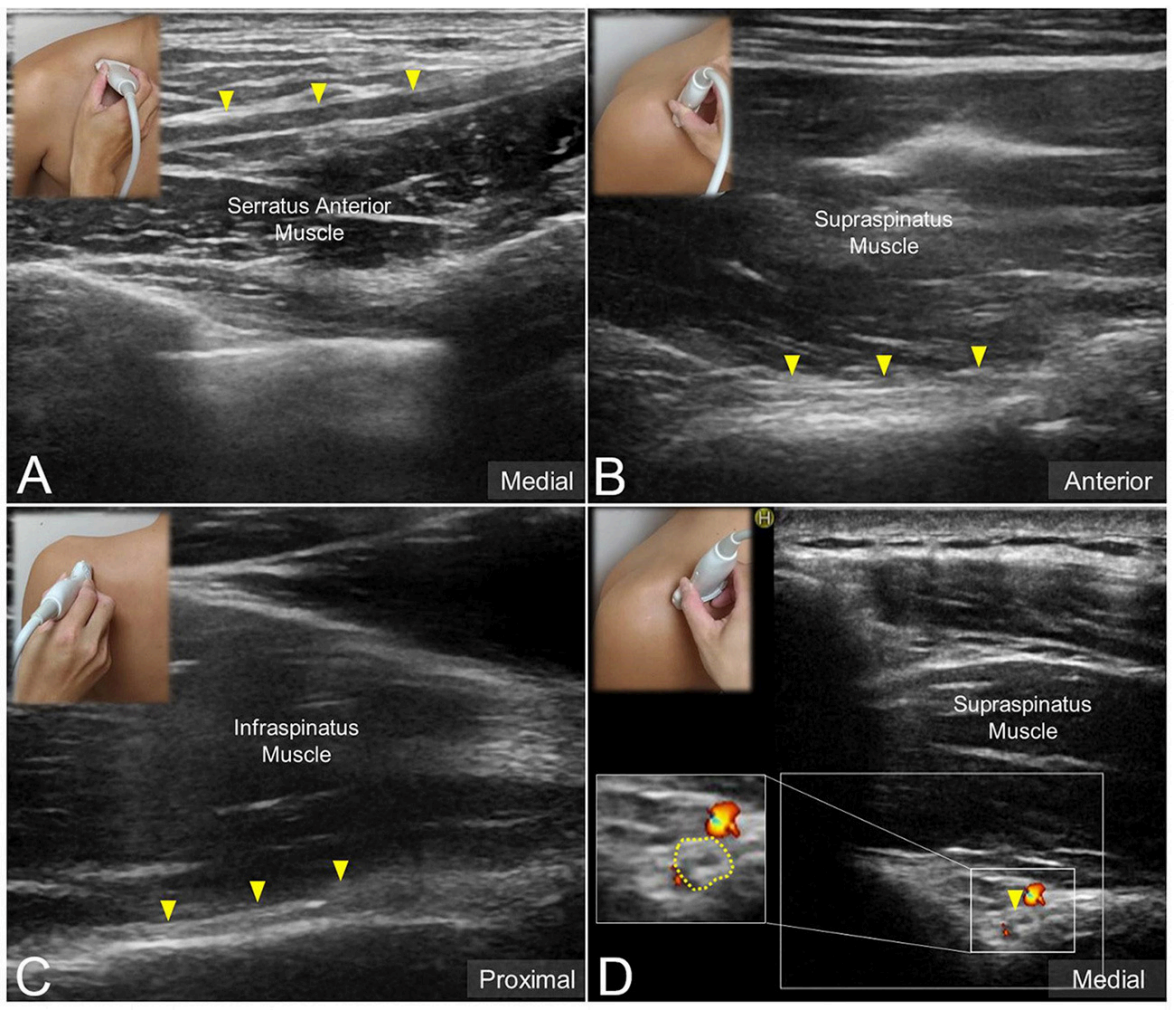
Ultrasound imaging of the suprascapular nerve *(solid yellow arrowhead)* in the long axis at (A) the supraclavicular region, (B) the supraspinatus fossa, and (C) the infraspinatus fossa. Ultrasound Doppler imaging of the suprascapular nerve at the supraspinatus fossa (D). Dashed line: the border of the nerve sheath.
